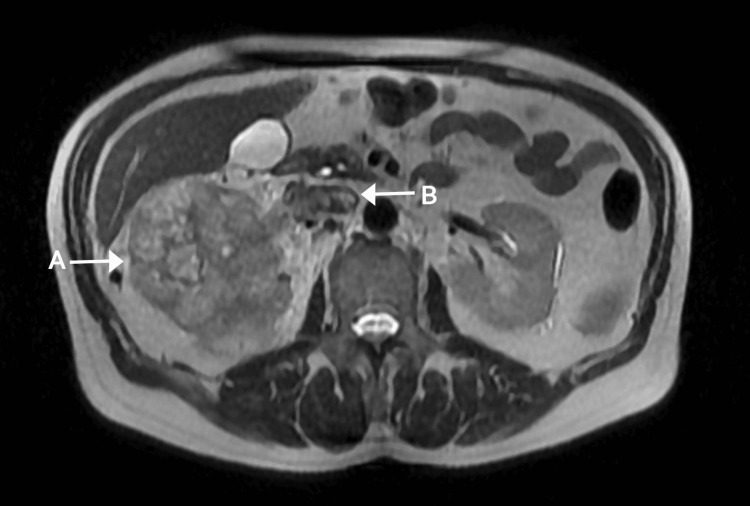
Axial section of angiographic MRI. Arrows: A: renal tumor, B: tumor thrombus.
